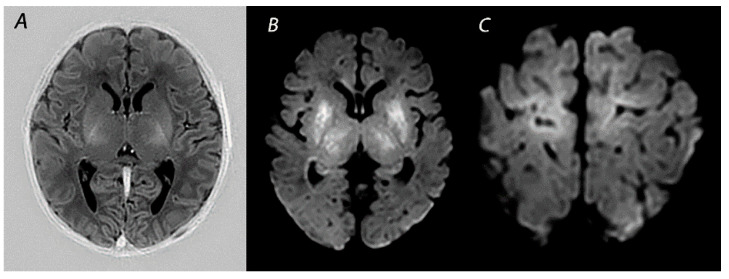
MRI performed on day 5 in a newborn born with Apgar scores 0/2/5 who received therapeutic hypothermia for HIE. Although the signal intensity of the posterior limb of the internal capsule appears normal on T1-weighted imaging (A), DWI shows extensive diffusion restriction in the basal ganglia and thalami (B), and the perirolandic cortex (C). The infant died after redirection of care.
